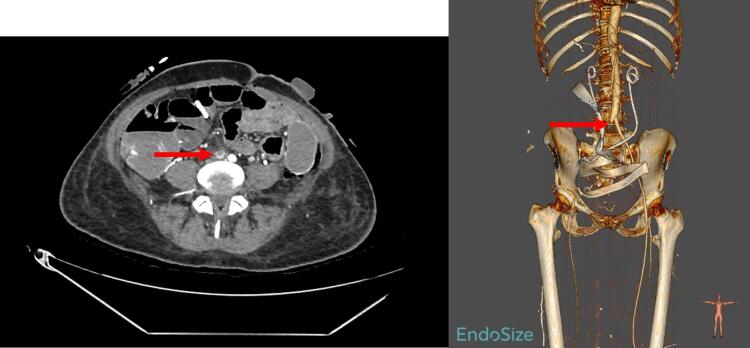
Contrast enhanced CT imaging at diagnosis, showing the right common iliac thrombosis and 3D reconstruction (red arrow).
